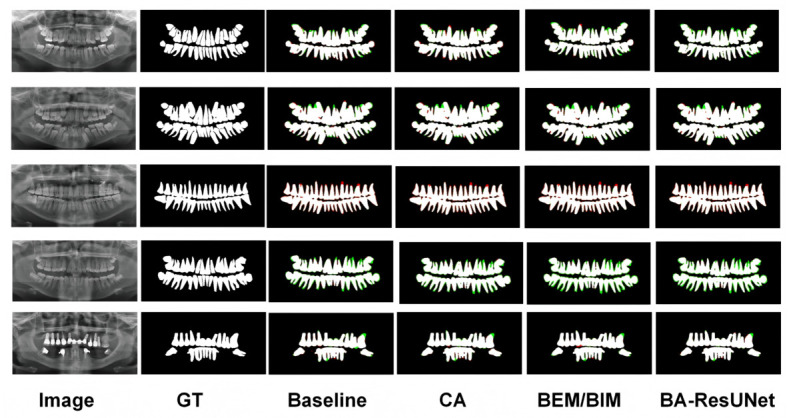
Qualitative ablation comparison of different model variants on panoramic dental X-ray images.
